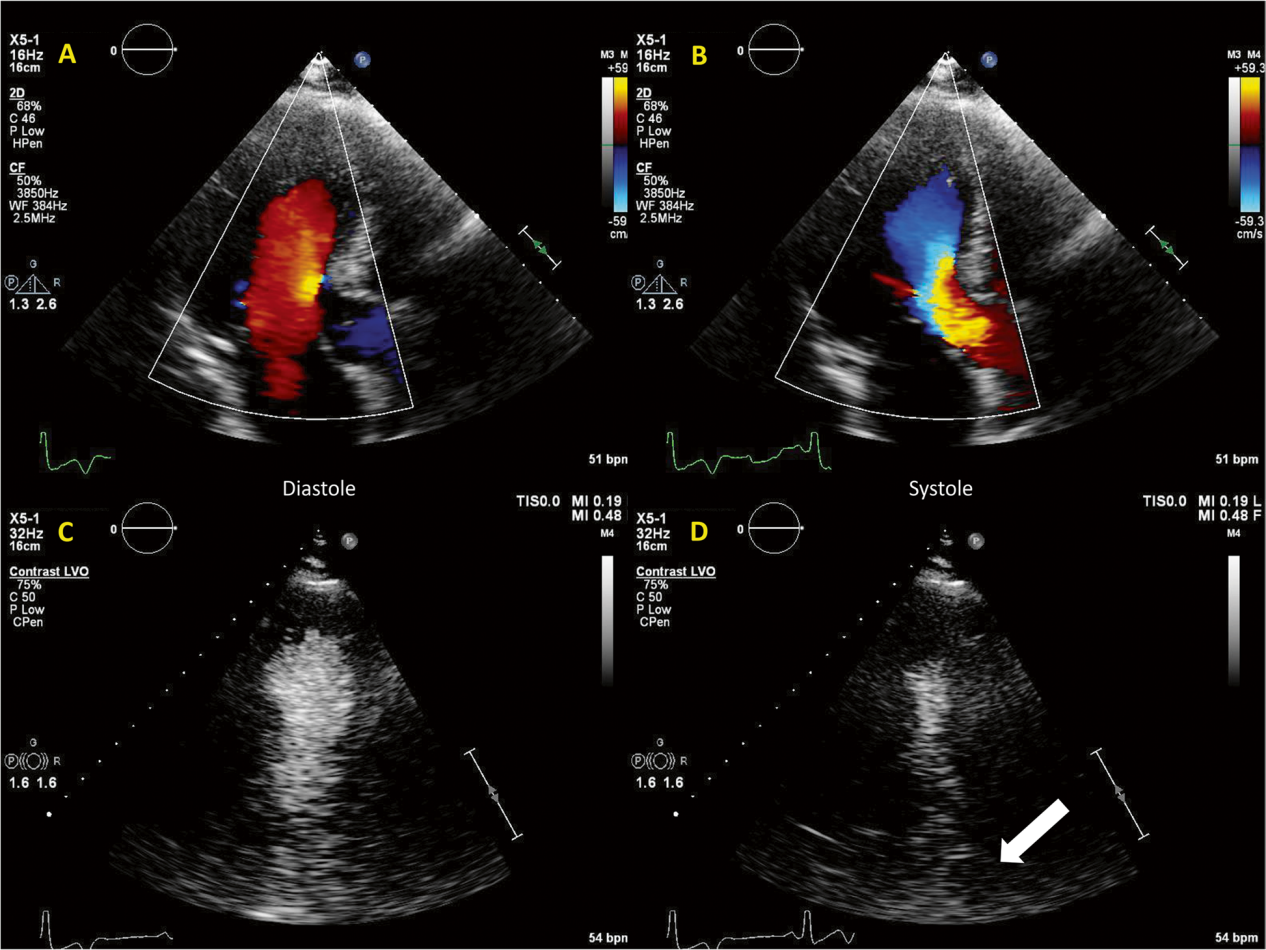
Patient 1. A, B: color Doppler (Additional file 2). There is no visible abnormal flow acceleration in the LVOT; C, D: Classical LV contrast imaging (Additional file 3) suggested cavity collapse towards LVOT (arrow)
